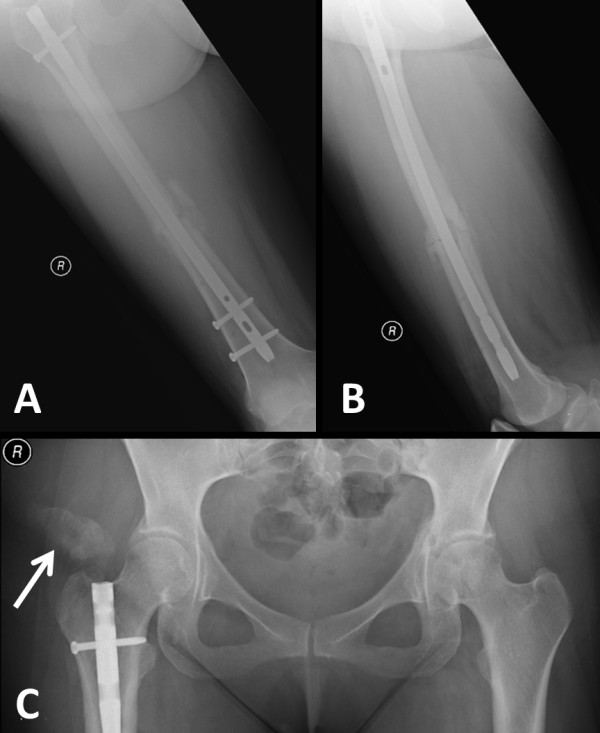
Evidence of fracture healing of the right femur fracture (A,B) and the nonoperative contralateral acetabular fracture (C) at two months of follow-up. The pelvic X-ray reveals early signs of heterotopic ossification in the soft tissues at the entry site of the femoral nail (arrow in C).
